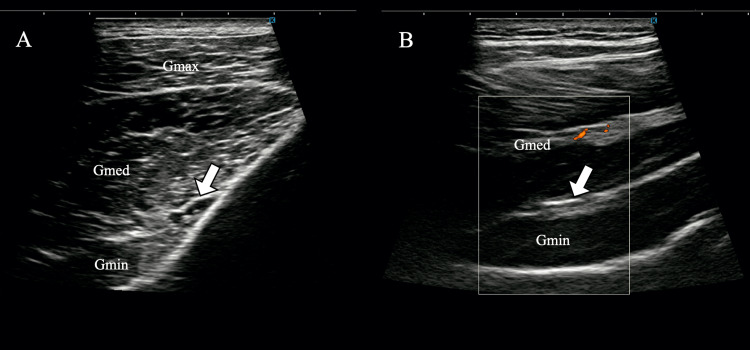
Tender points of the superior gluteal nerve identified under ultrasound guidance (A) Superior gluteal nerve near the suprapiriform foramen. (B) Inferior branch of the superior gluteal nerve, located between the gluteus medius and minimus. Arrows indicate the superior gluteal nerve. Gmax, gluteus maximus; Gmed: gluteus medius; Gmin: gluteus minimus.
